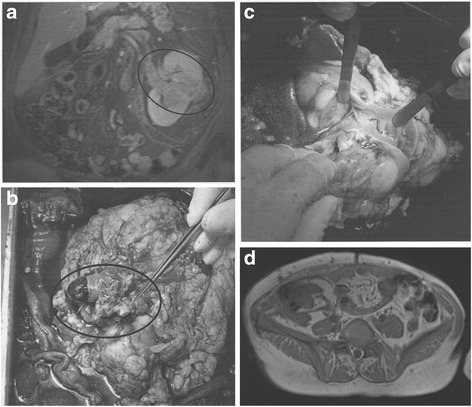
a Initial MRI scan PADUA 12p. b Tumor thrombus occupying the renal vein. c Back-table tumor resection and renal reconstruction. d MRI scan showing tumor-recurrence laterally in the autotransplanted kidney in the right iliac fossa. a–d MRI-images and photographs demonstrating a rare case of an extensive clear cell renal cell carcinoma of the lower pole, extending into the renal hilum and forming a venous tumor thrombus in the inferior vena cava grades PADUA 12p (a). A radical nephrectomy, cavotomy, and tumor-thrombectomy were performed (b). In ice water, a tumor resection and renal reconstruction was undertaken (c) followed by autotransplantation. Local recurrence occurred at 48 months, and a partial nephrectomy of the autotransplanted kidney was undertaken. Finally at 105 months, a nephrectomy was performed for a second recurrence (d). At 42 months after nephrectomy, a systemic therapy was introduced for metastases
